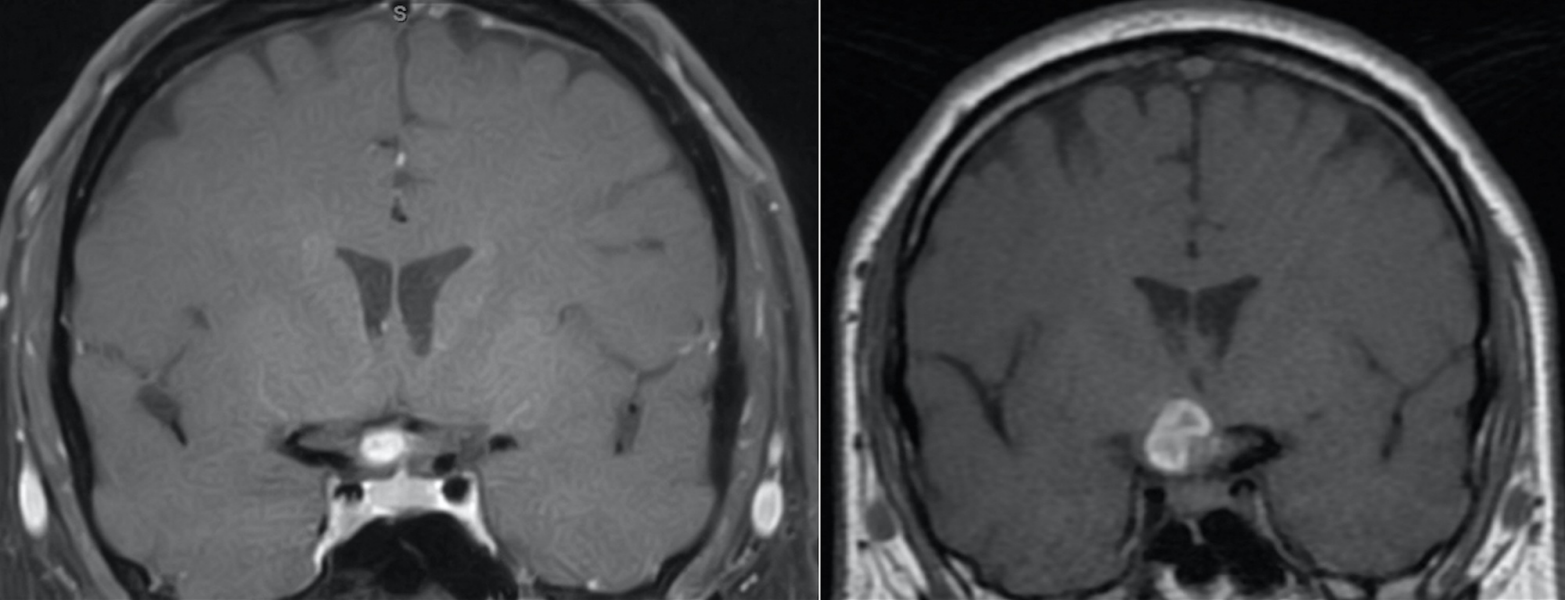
Coronal T1-weighted contrast MR image (left) showing hyperintensity along the right optic chiasm and (right) increase in size two months later, consistent with hemorrhagic CM MR: magnetic resonance; CM: cavernous malformation
